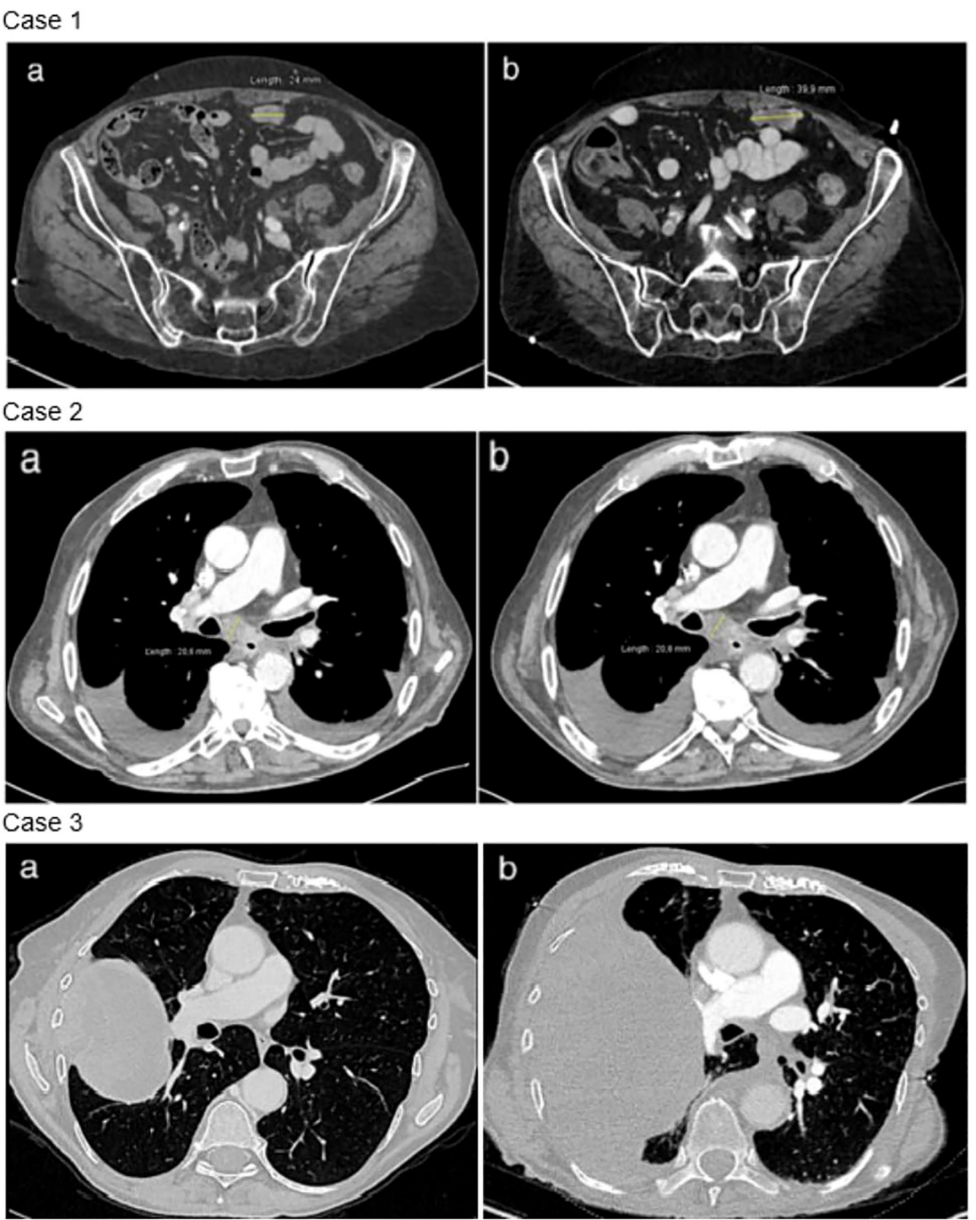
Radiological evolution under trametinib. Case 1: disease progression between baseline thoraco-abdominal computed tomography (a) and after 7 weeks of trametinib (b). Example of a peritoneal metastasis. Case 2: stable disease between baseline thoraco-abdominal computed tomography (a) and after 6 weeks of trametinib (b). Case 3: disease progression between baseline thoraco-abdominal computed tomography (a) and after 8 weeks of trametinib (b).
